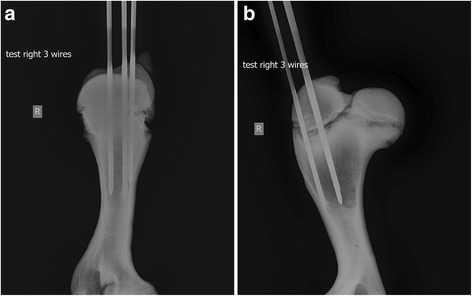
Orthogonal cranio-caudal (a) and latero-medial (b) projection radiographs of a prototype pre-test bone. Note the cortical engagement that was not avoided in this prototype test
